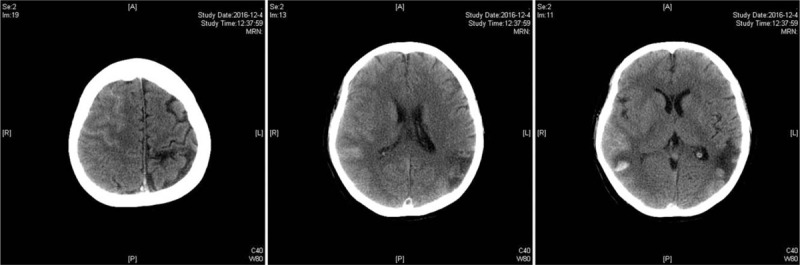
On admission, a CT scan revealed linear high-density images in the partial gutter of the right frontal, parietal, and occipital lobes, as well as patchy high-density images in the right parietal and left temporal lobes. CT = computed tomography.
